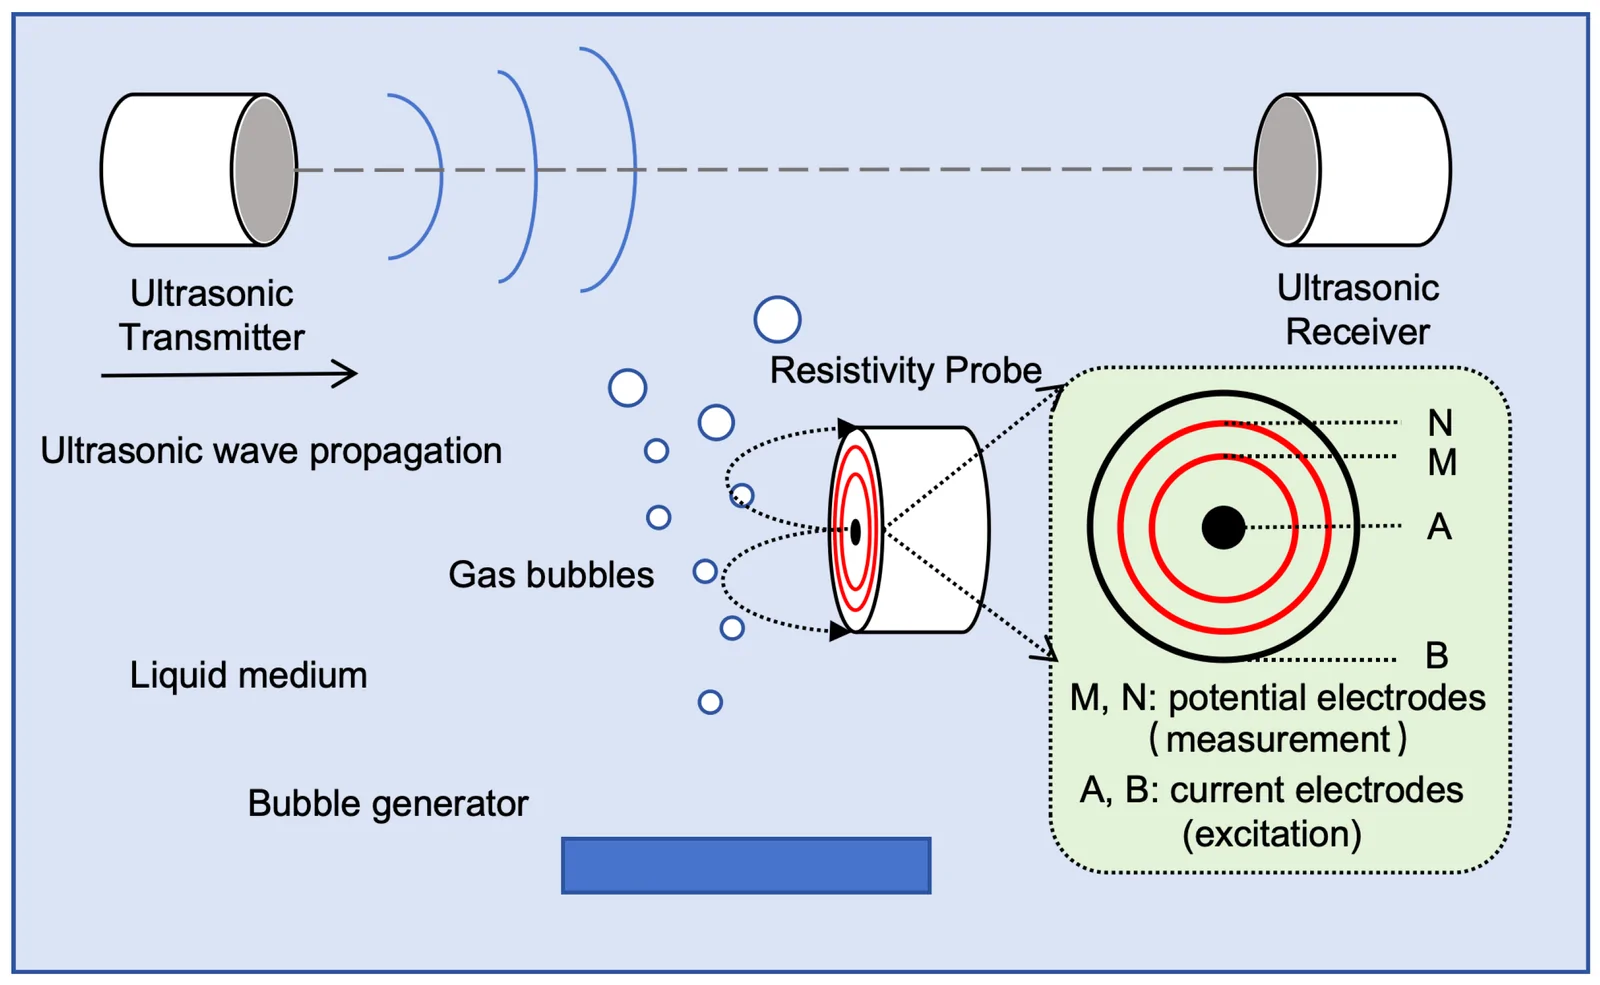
Sensing principles of the ultrasonic transmission channel and the resistivity probe. Both sensors monitor the same vertical section of the static column but respond over different spatial regions.
